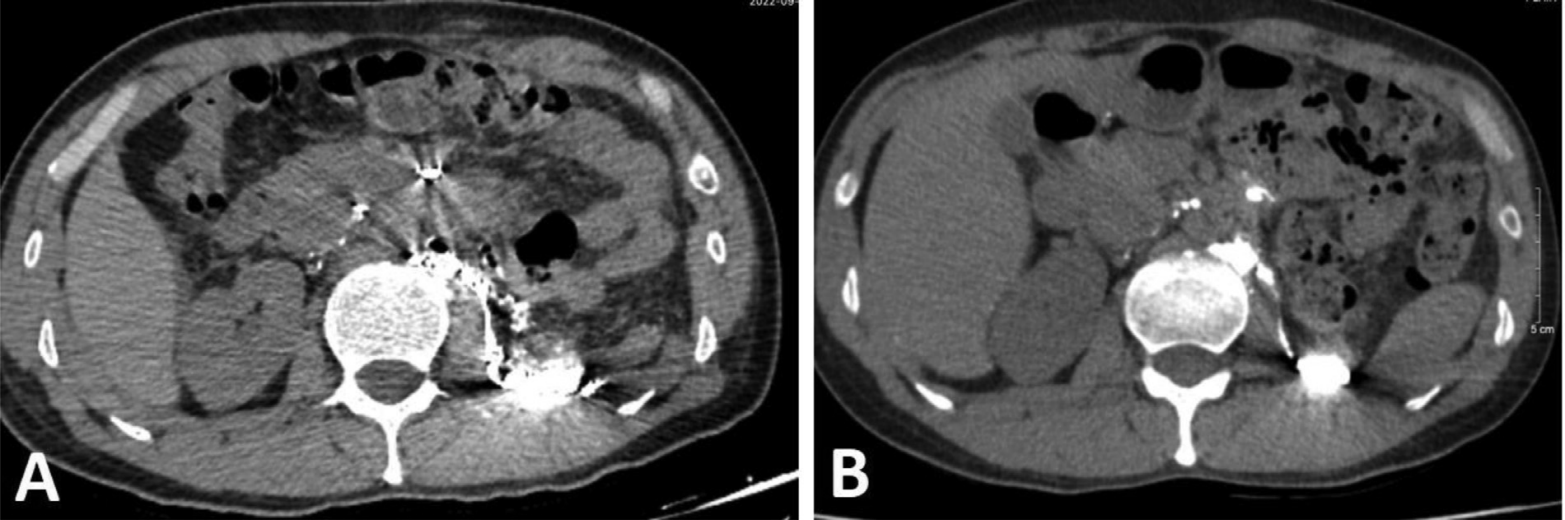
Abdomen CT scan after intervention (A) and 3 months after discharge (B) showed deposition the glue cast of the lymphatic vessel and the left renal fossa.
